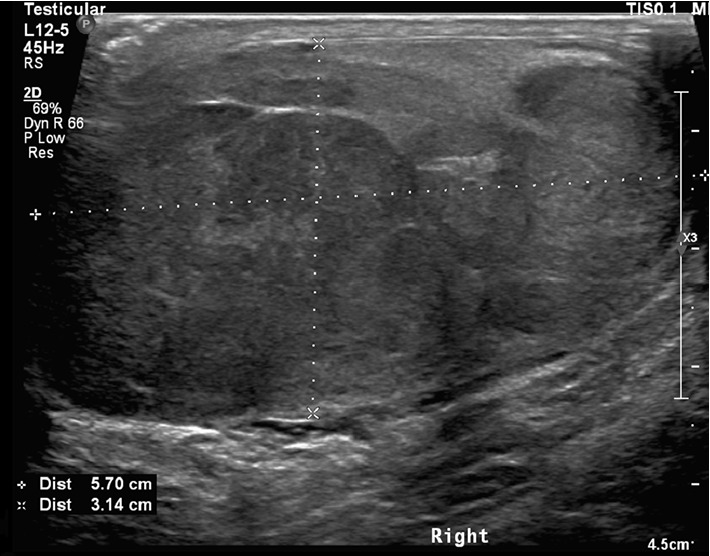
Ultrasound of right testicle.
